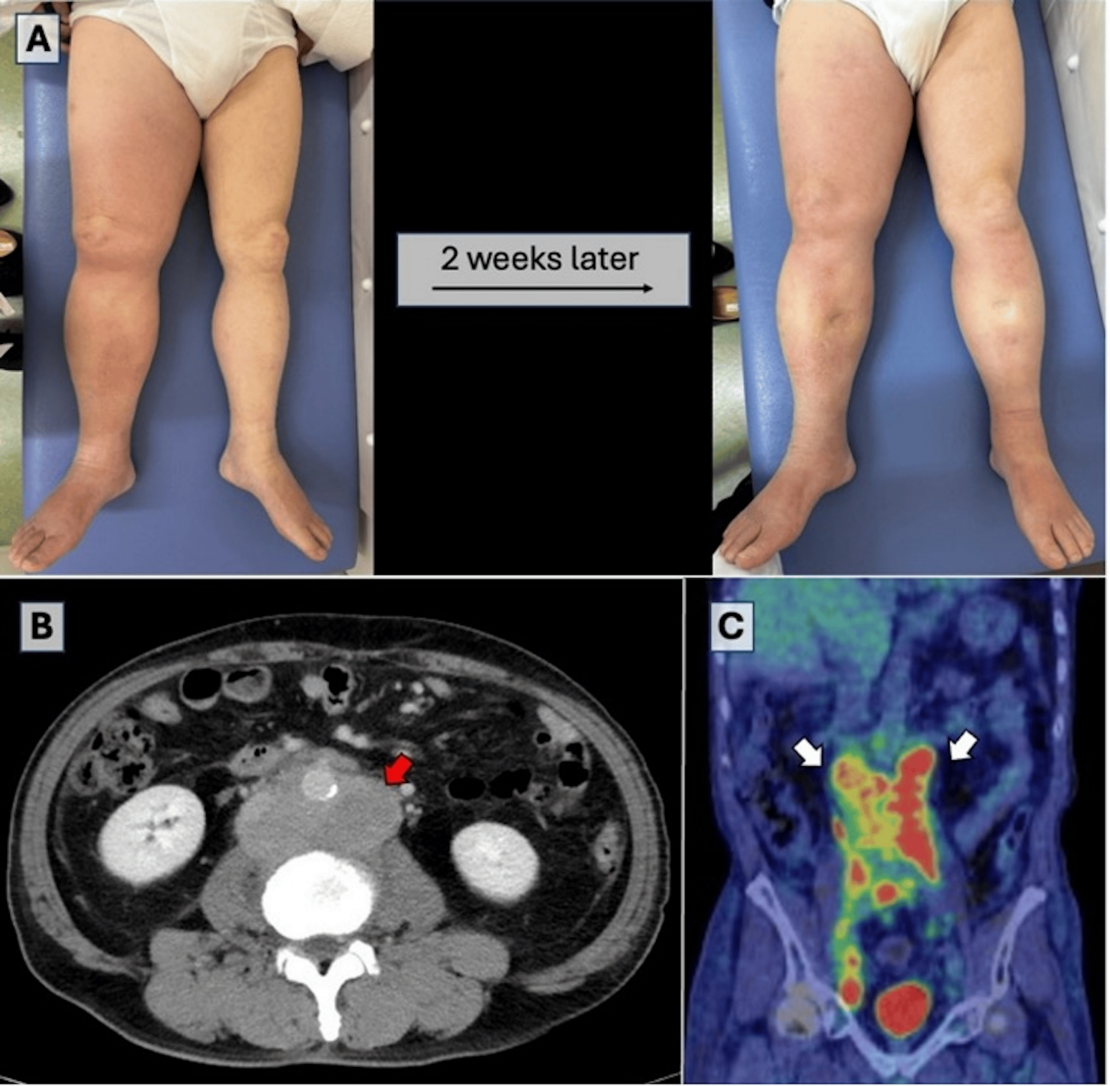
Clinical course of leg edema, abdominal/pelvic CT, and FDG-PET/CT A: leg edema progresses from unilateral to bilateral over two weeks; B: abdominal/pelvic contrast-enhanced CT in the axial view shows enlarged lymph nodes around the paraaortic lesion (red arrow); C: FDG-PET/CT in the coronal view shows increased uptake in multiple enlarged lymph nodes (white arrows). FDG: fludeoxyglucose-18; PET/CT: positron emission tomography/computed tomography
